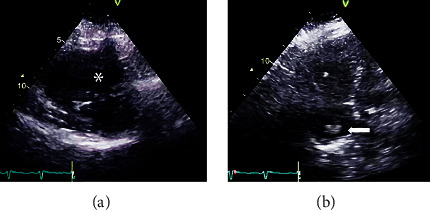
Echocardiography before thrombolysis showed severe right ventricular dysfunction with flattened intraventricular septum (^*∗*^) in parasternal short-axis view (a) and thrombus (arrow) in the right atrium in apical four-chamber view (b).
